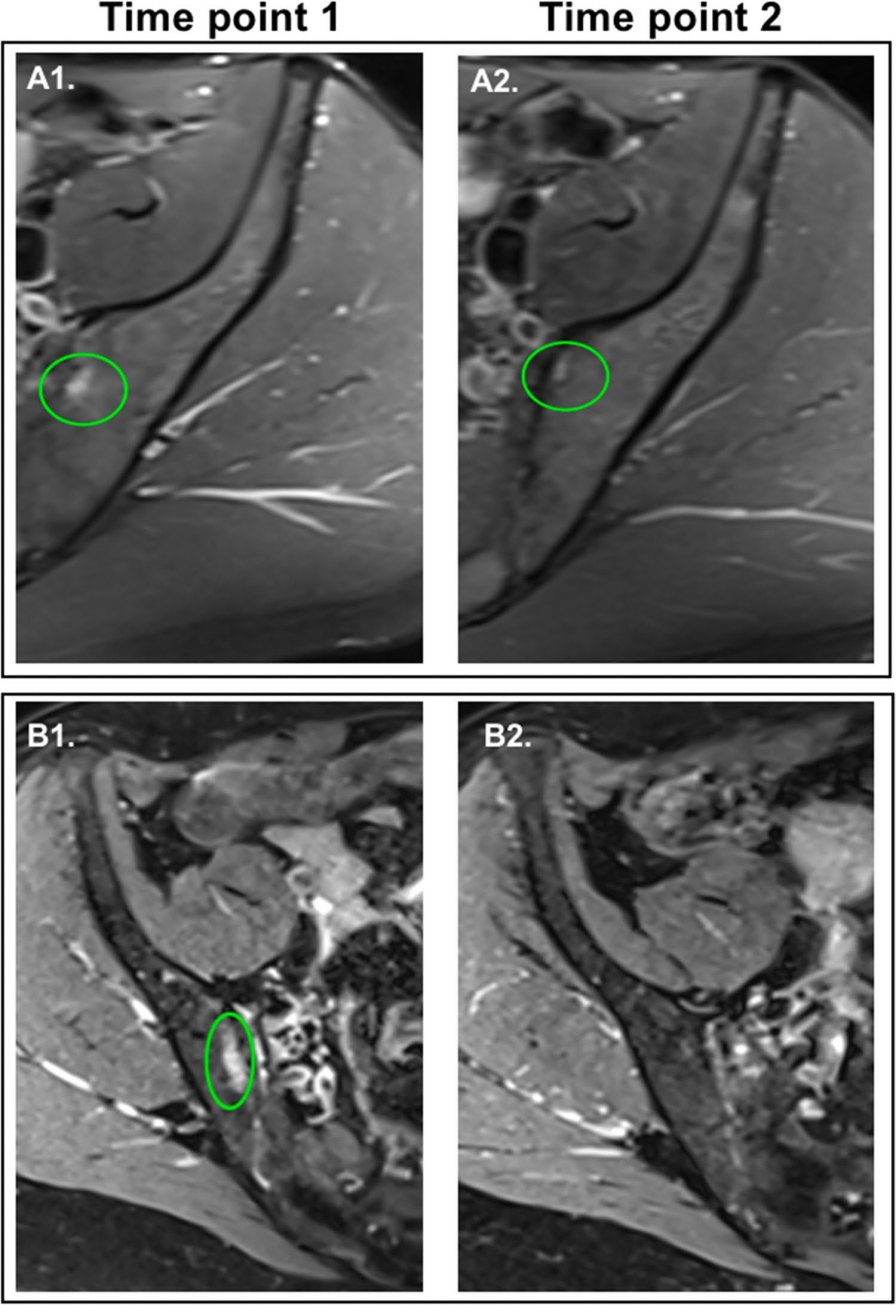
Axial Dixon PD FS TSE MR images of 2 participants: A one showing subchondral oedema in the iliac side of left sacroiliac joint at time point 1 (A1) which got smaller in size at time point 2 (A2) in a 35-year-old man; B one with oedema in the iliac side at time point 1 (B1) which disappears at time point 2 (B2) in a 58-year-old woman
